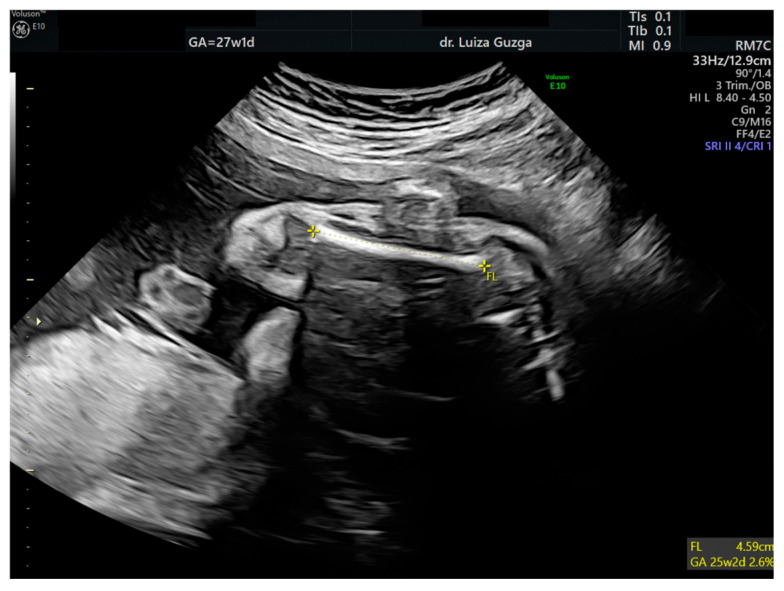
Two-dimensional ultrasound assessment of the fetus at 27 weeks of gestation, identifying a short femur. Abbreviations: FL (femur length); GA (gestational age); w (weeks); d (days).
